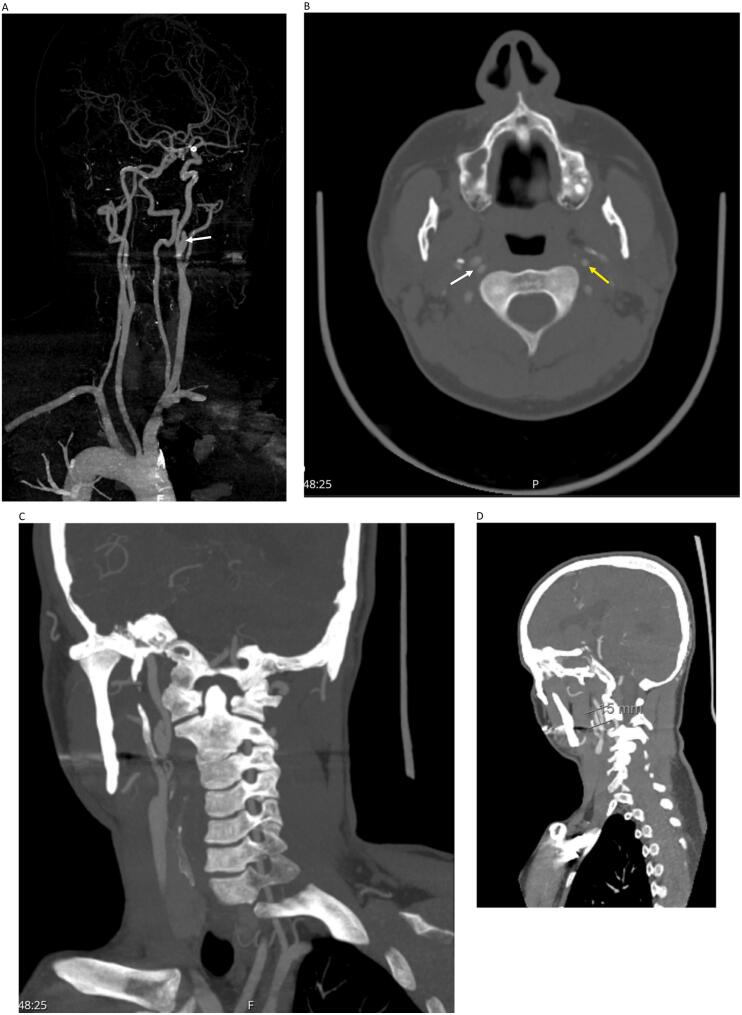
A: 3D reconstruction showing persistent right ICA pseudoaneurysm (arrow). B: CT angiography axial section showing normally appearing left ICA (yellow arrow) and dissected right ICA (white arrow). C: Maximum intensity projection showing right ICA pseudoaneurysm. D: Sagittal reconstruction with pseudoaneurysm size 15 mm.
